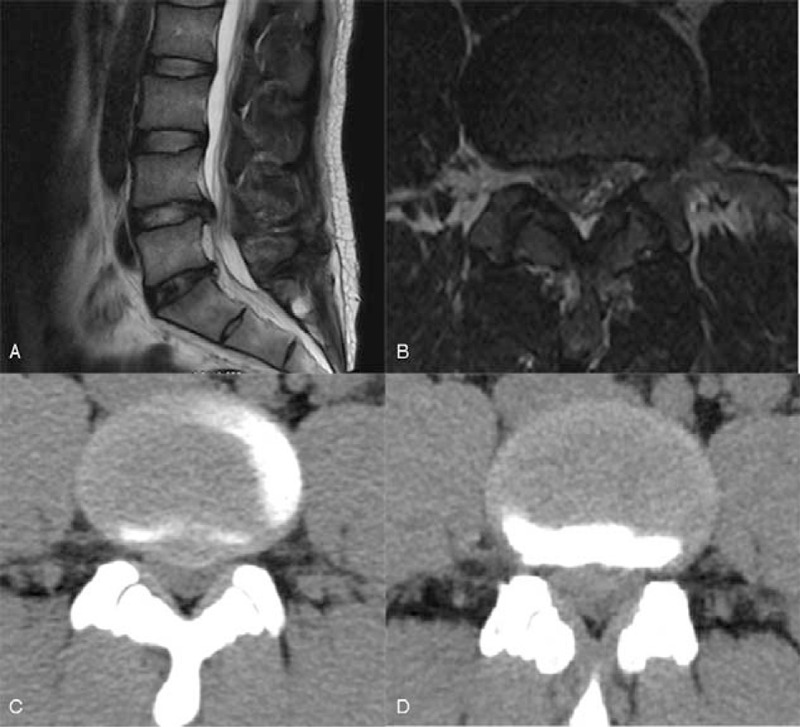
Preoperative CT and MR imaging showed central to right sided disc herniation at the L4/5 level.
